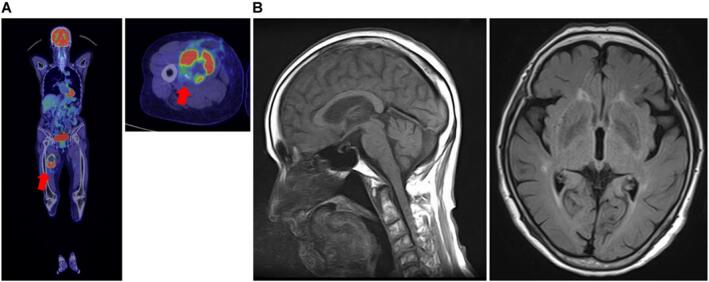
Preoperative general examination. (A) Positron emission tomography-computed tomography scan did not show abnormal accumulation except in the right thigh (SUVmax: 14.1). (B) T2-weighted FLAIR image of brain MRI showed no neoplastic lesions.The only neoplastic lesion was in the right thigh, and there was no tumor in the central nervous system region.
